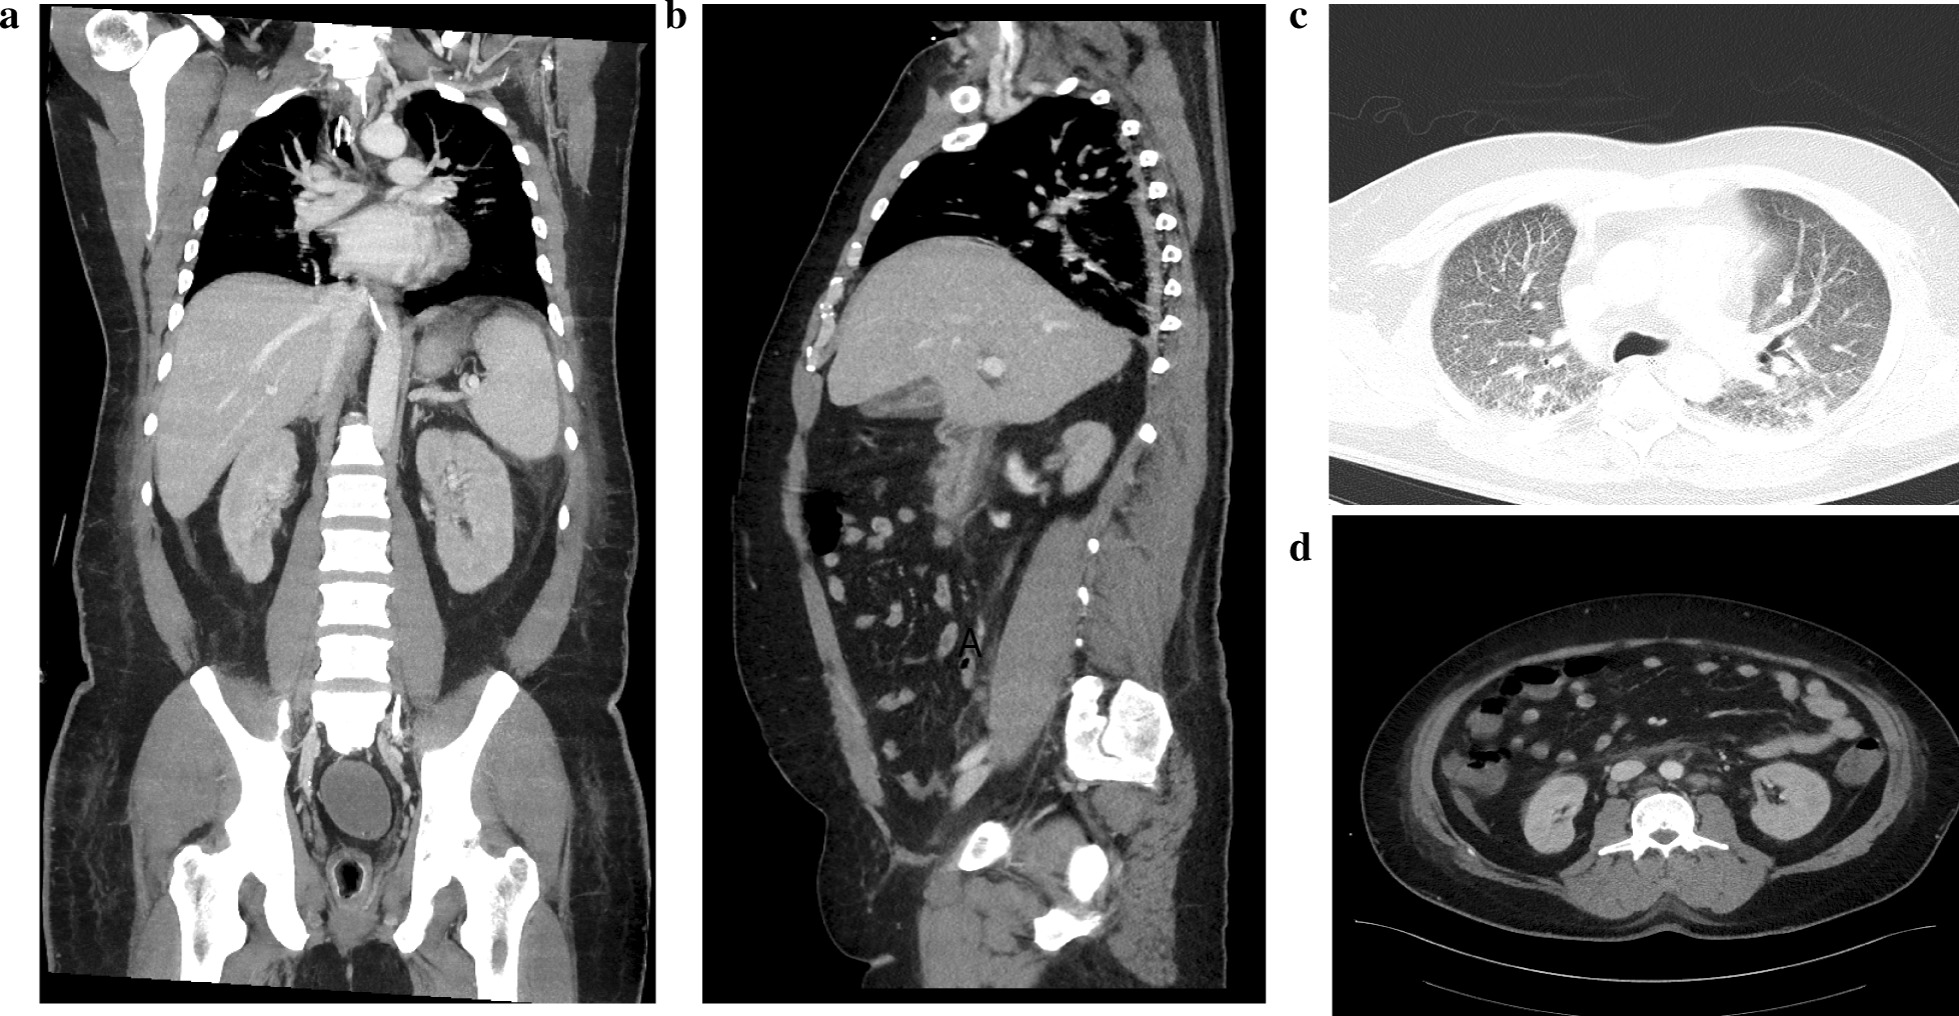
Radiological examination of the patient. Computed tomography scan images of the abdomen taken at admission demonstrating enlarged spleen and liver, lymphadenopathy in the abdomen, thickened wall of colon and bile duct, and atelectasis in the lung sections
